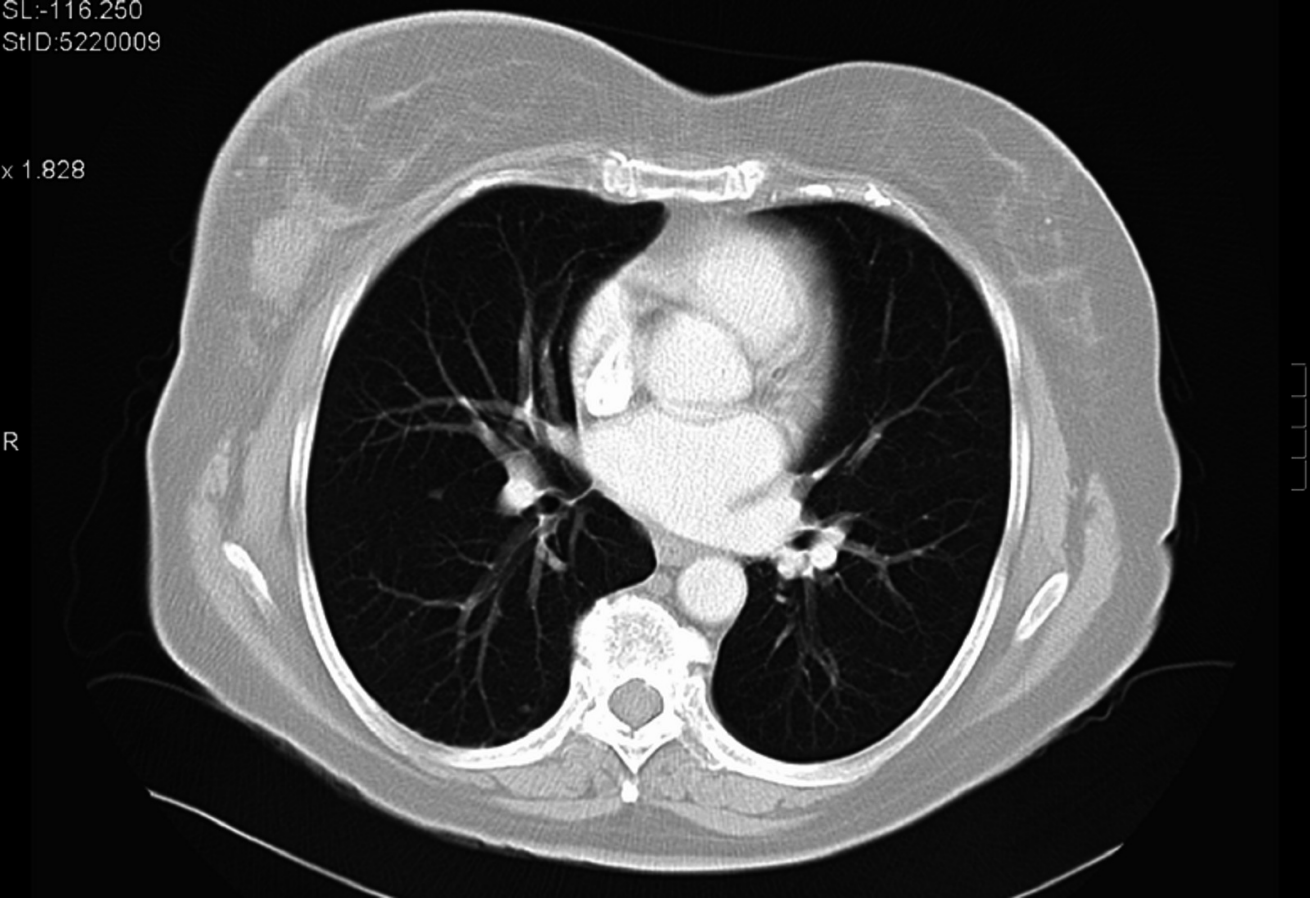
Patient 3: Computed tomography (CT) imaging reveals lung nodules pre treatment
